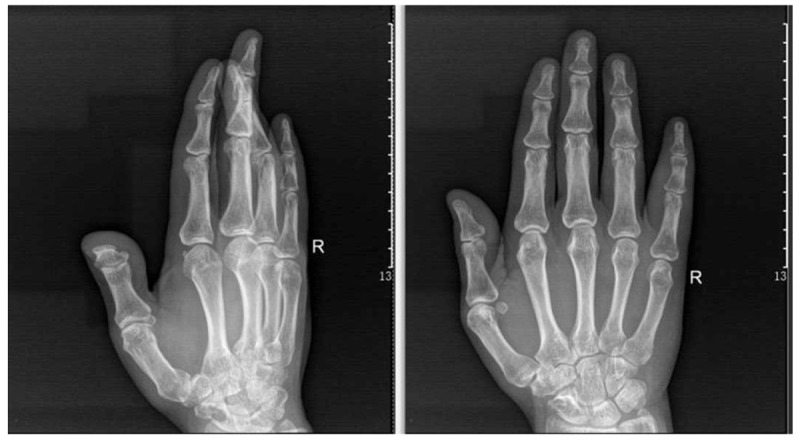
Soft tissue swelling in little finger in x-ray.
